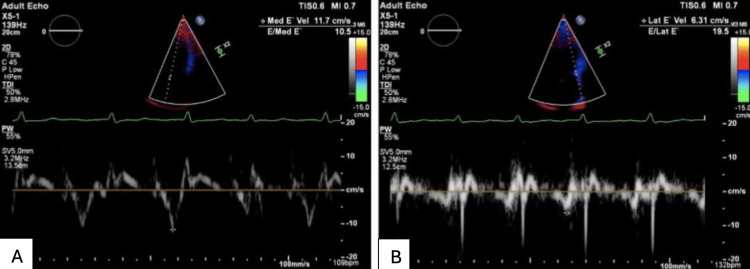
Transthoracic echocardiogram (apical four-chamber view) showing tissue Doppler imaging of medial (A) and lateral (B) mitral annular early diastolic velocities (e′), demonstrating annulus reversus (medial e′ > lateral e′), consistent with constrictive pericardial physiology.
